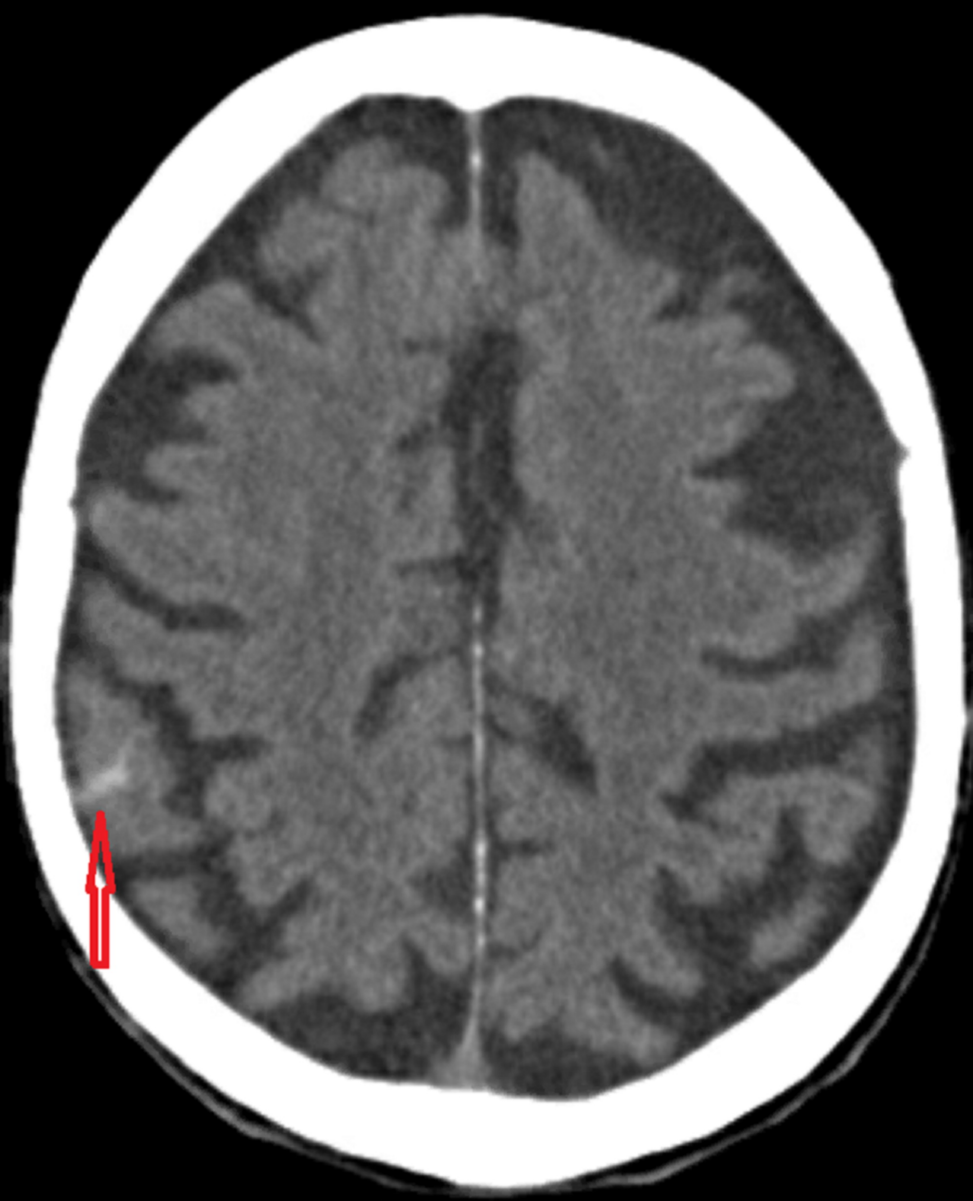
Noncontrast computed tomography scan of the head, axial view showing small focus of subarachnoid hemorrhage (arrow). No evidence of midline shift.
